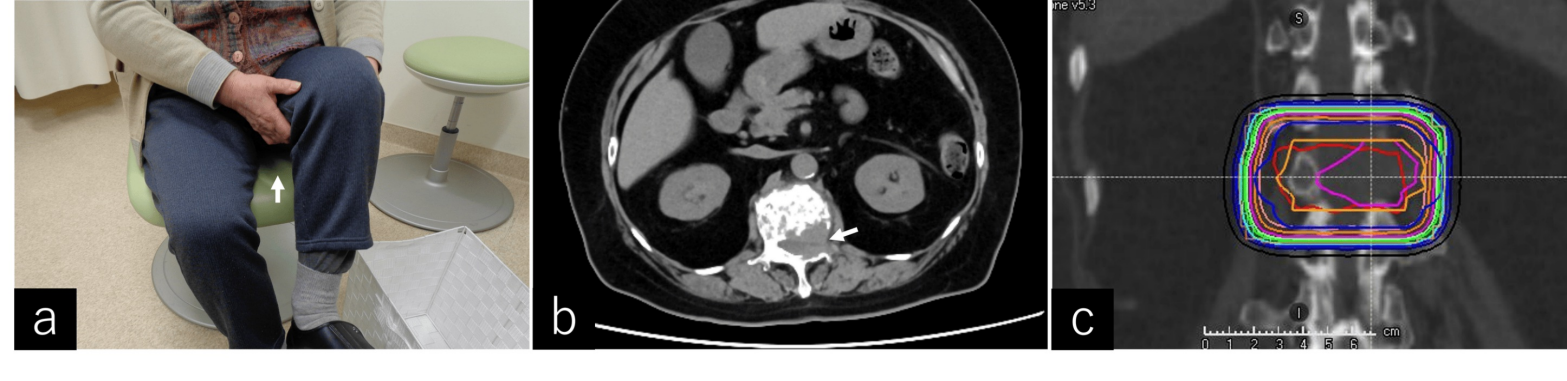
Case 3: Ishikawa-Teramura sign, CT imaging of lumbar spine tumor invasion, and radiation therapy planning. Ishikawa–Teramura Sign (IT sign) (a). CT of the lumbar spine revealed tumor invasion of the 1st lumbar vertebra (arrow) with no evidence of significant invasion into adjacent structures (b). Radiation therapy (RT) planning involved irradiation from two fields in the anterior-posterior direction. The patient received 36 Gy in 12 fractions of RT for the 1st lumbar vertebra (c).
